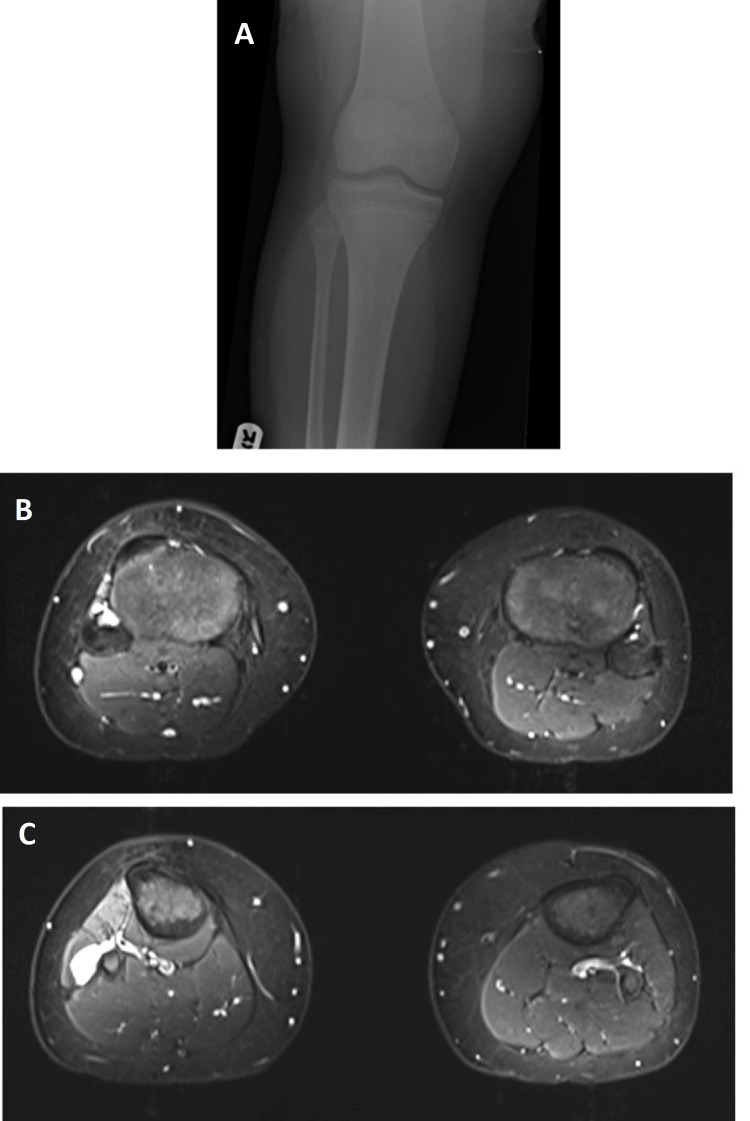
(A) Plain radiographs of the right knee – no bony abnormality seen. (B, C) Axial cuts in T2-weighted MRI images of both legs at the level of proximal tibia showing right cystic lesion in close relation to the CPN and the proximal tibiofibular joint. CPN - common peroneal nerve
